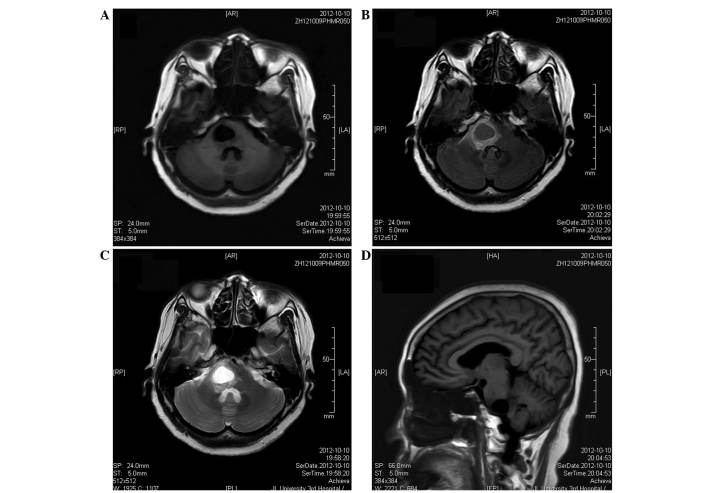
Magnetic resonance images obtained three months following the initial gamma knife radiosurgery. The cystic mass increased in size to 22×17×19 mm (volume: 3.9 cm^3^). (A) Axial T1-weighted image; (B) axial T2-weighted, fluid-attenuated inversion recovery image; (C) axial diffusion-weighted image; and (D) sagittal T1-weighted image.
